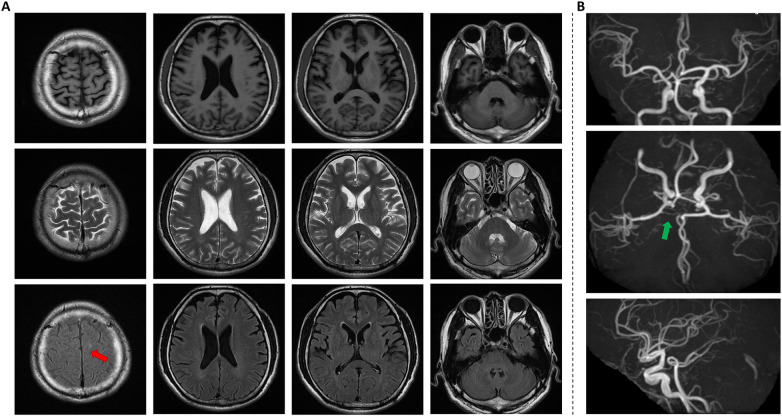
Cranial MRI + MRA findings of the patient. (A) Axial images of 4 representative slices (upper: T1-weighted, middle: T2-weighted, lower: T2-FLAIR), which revealed punctate white matter degeneration in the left frontal lobe (red arrow). (B) Multi-angle MRA indicated that the A1 segment of the right anterior cerebral artery was slender, suggestive of a developmental variant (green arrow). No imaging features of common etiologies of secondary cluster headache were observed, including pituitary adenoma, cerebral arteriovenous malformation, internal carotid artery aneurysm, and cerebral venous sinus thrombosis.
